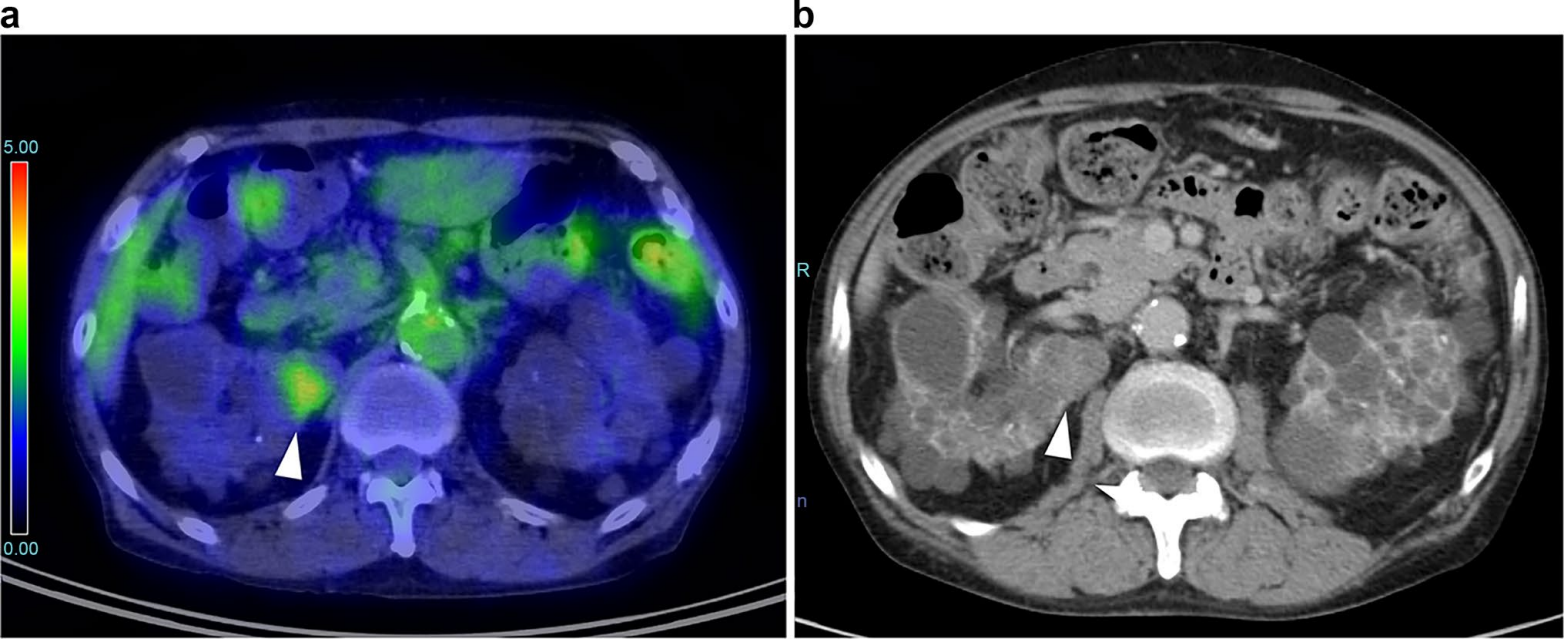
68-year-old man with surgically-proven ACD-RCC. a Axial FDG-PET/CT shows a focal accumulation in the right kidney with an SUV_max_ of 3.3 (arrow). b Contrast-enhanced CT shows a right renal mass, consistent with the accumulation showing weak enhancement (arrow)
